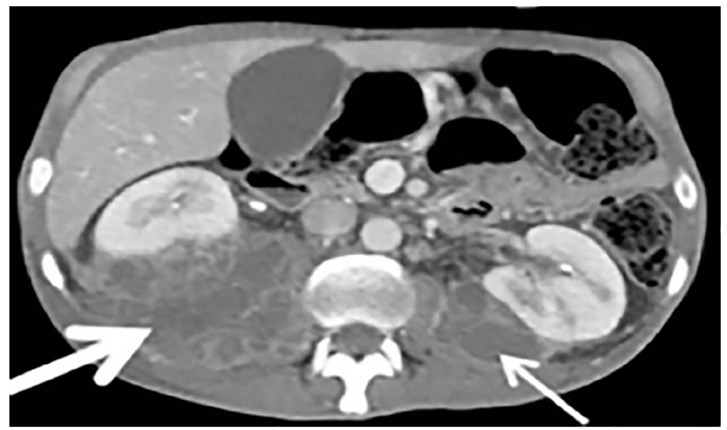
Computed tomography with intravenous contrast demonstrates bilateral psoas abscesses. The right-sided abscess is much larger as indicated by the thicker arrow.
